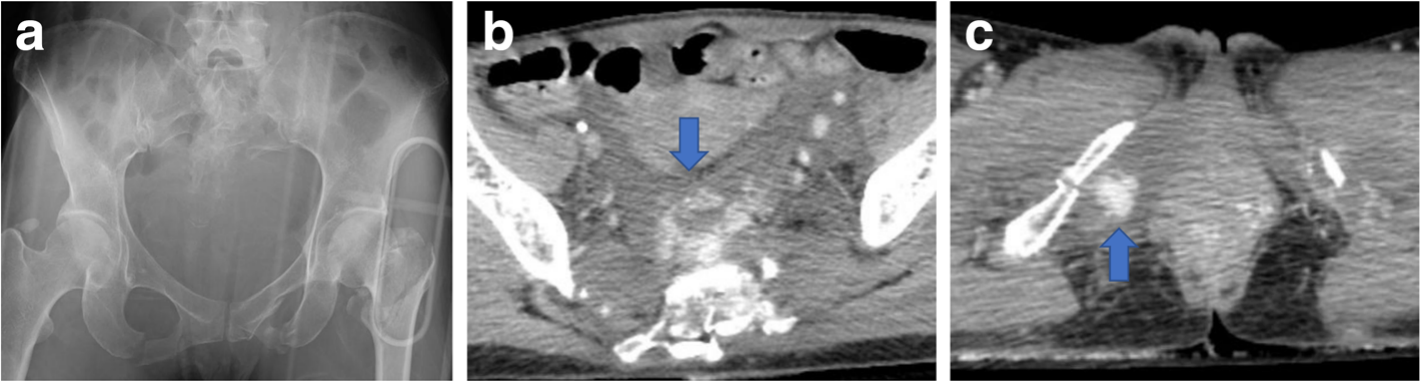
Pelvis X-ray (a) and contrast computed tomography (b and c) at the initial arrival. a Fractures of pubic bones, hipbones on both sides, and sacral bones are seen and there are unstable pelvic fractures. A massive retroperitoneal hematoma and extravasation of the contrast media on the anterior of sacrum (b; arrow) and right perineum (c; arrow) are recognized
